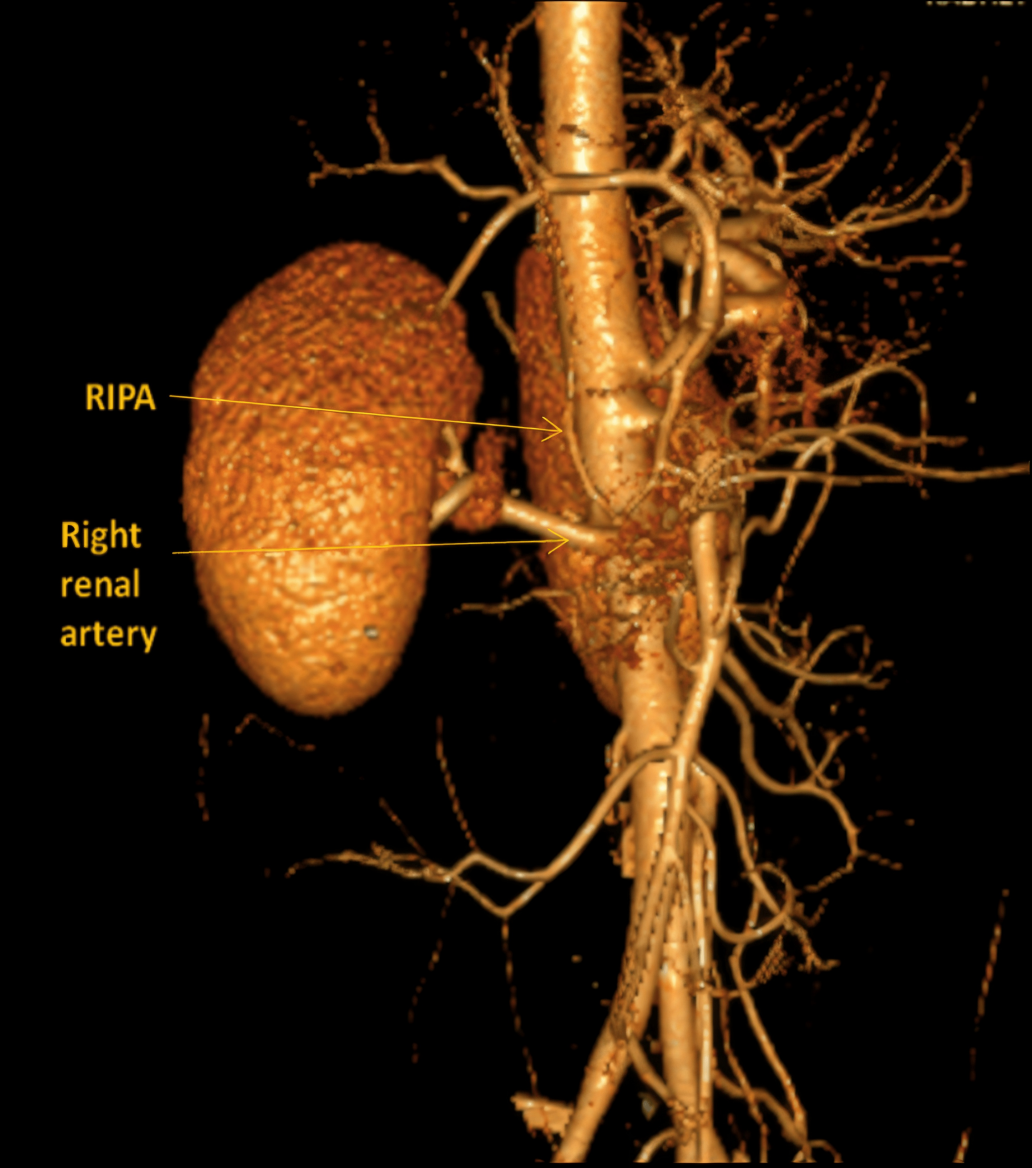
Volume-rendered CT image showing origin of RIPA from the aorta below the origin of the SMA RIPA, right inferior phrenic artery; SMA, superior mesenteric artery
